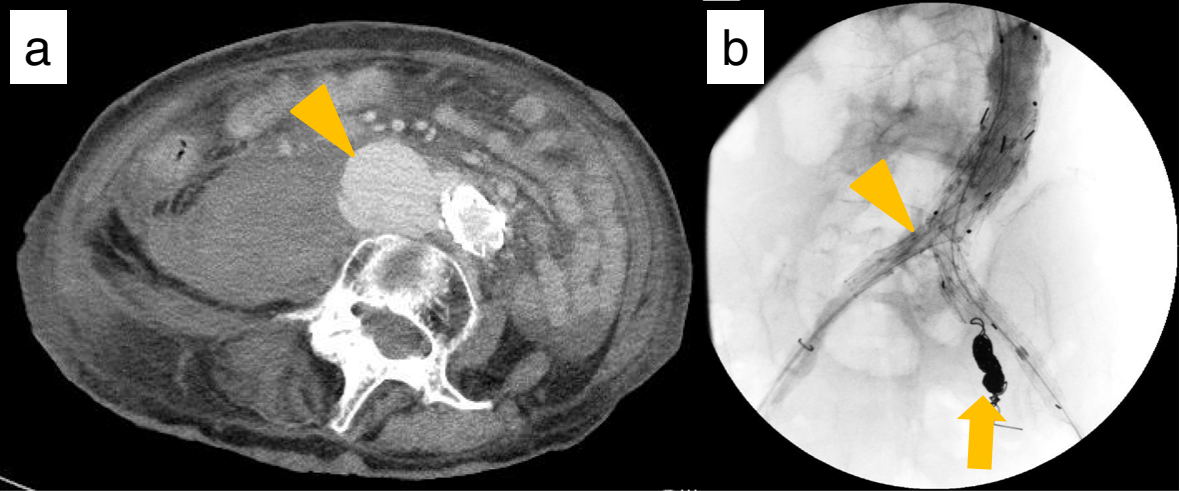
Images of the abdominal aortic aneurysm before and during the secondary operation. a An abdominal contrast CT demonstrated type Ib endoleak (orange arrowhead). b An AFX stent and infrarenal cuff (orange arrowhead) were deployed, and a coil embolization performed of the left internal iliac artery aneurysm (orange arrow)
